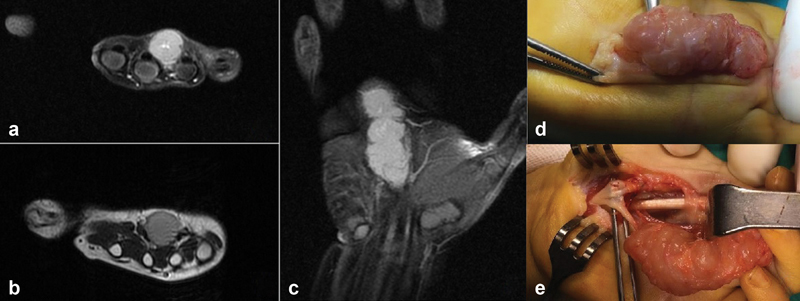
a,b,c) an MRI imaging, the lesion is approximately 4 × 1.5 × 2 cm in size, located between the 3rd and 4th metacarpals of the left-hand volar face, adjacent to the flexor tendon, hypointense in T1 sequence, hyperintense in T2 sequence. d,e) on intraoperative images, the lesion appears to involve the lateral branch of the median nerve.
